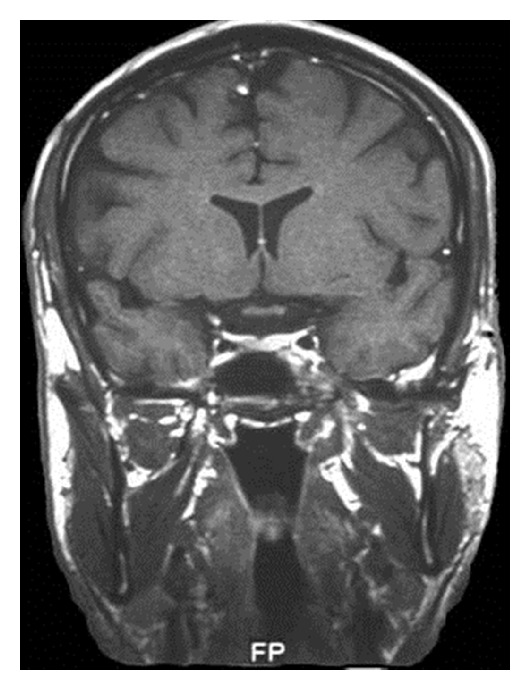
Cranial MRI 16 months after radiotherapy.
